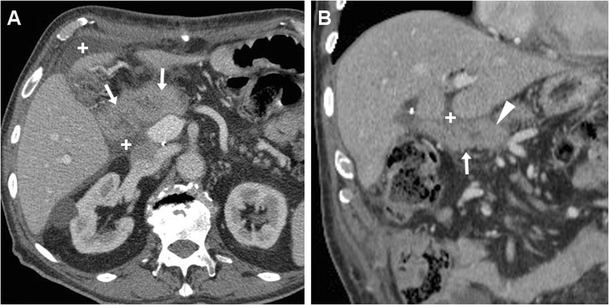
a, b Transient oedema of the MJL after uncomplicated PD in a 72-year-old man. Contrast-enhanced CT showed mildly thickened MJL walls (*arrows*). Note PR (*arrowhead*), minimal subhepatic fluid (+)
